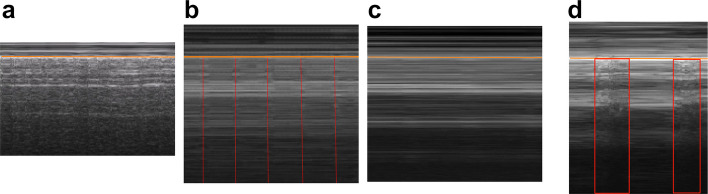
Representative M-mode lung ultrasound patterns demonstrating the four relevant classes of pleural motion (a to d from left to right). Horizontal “orange” line: pleural line. a Lung sliding (seashore sign) – normal lung motion pattern, ruling out PTX. b Lung pulse (T-lines sign) – indicates cardiac-induced pleural motion without respiratory sliding, also ruling out PTX. See vertical “red” lines originating from the pleural line. c Absence of lung sliding (barcode sign) – indicates lack of pleural motion, consistent with PTX. d Lung point – clear boundary between moving and non-moving pleural segments, pathognomonic for PTX. Vertical “red” boxes: normal motion with clear border with non-moving segments
